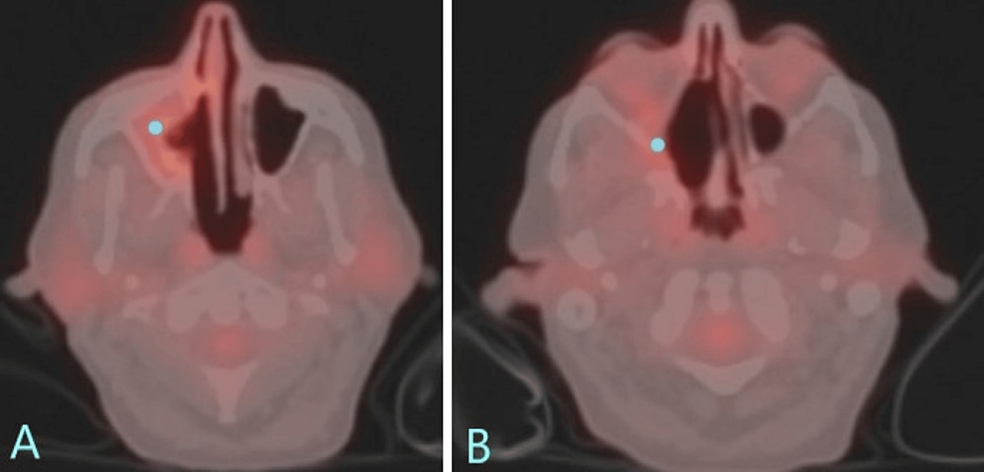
A three-month post-treatment PET-CT axial plane (A, B). Interval right frontoethmoidectomy and bilateral sphenoidectomy with tumor resection noted. A mild amount of mucosal thickening of the right maxillary sinus (blue dot), which was mildly FDG avid, with an SUV 3.4.
